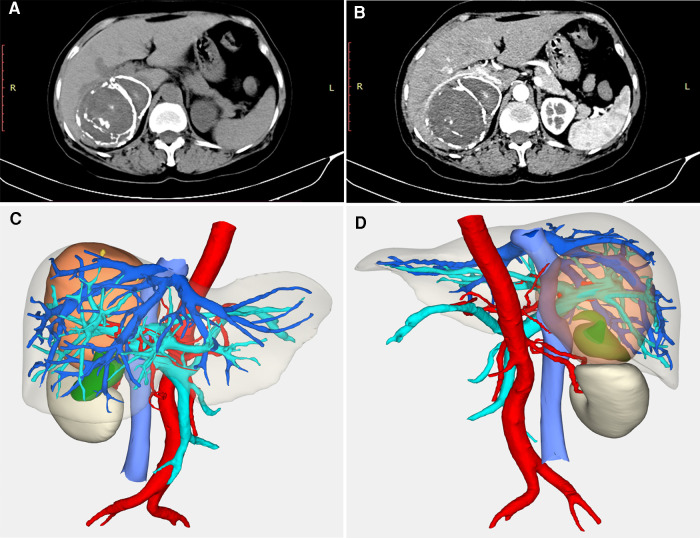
Imaging examination for case 2. (A) an “egg-shell” mass locating in the right subhepatic area; (B) the mass had a calcified edge wrapping around the homogeneous content with multipoint calcifications and calcified separations in the artery phase; (C) three-dimensional CT reconstructed imaging revealed that the right hepatic vein and hepatic pedicle were compressed from the dorsal to the ventral part (anterior); (D) three-dimensional CT reconstructed imaging (posterior).
